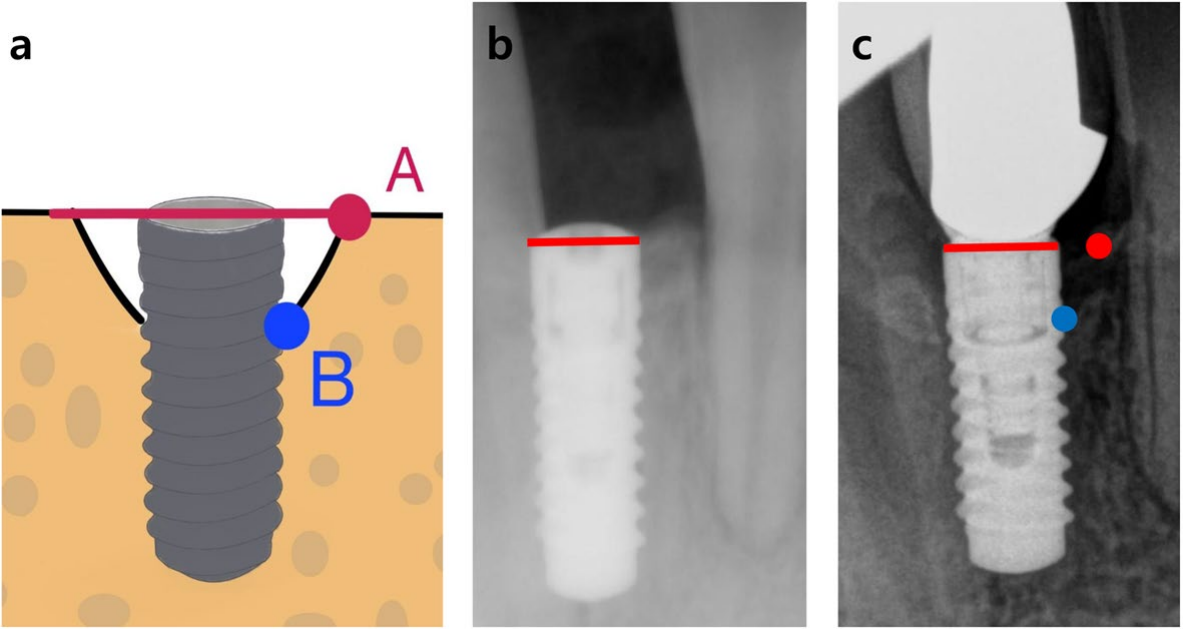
a Measurement of vertical and horizontal bone loss of peri-NDIs. A The point of first contact between the parallel extension line of the implant shoulder and the alveolar bone. B The contact point between the implant surface and the alveolar bone. b The postoperative clinical radiographs of NDIs. c The clinical radiographs of peri-implantitis
